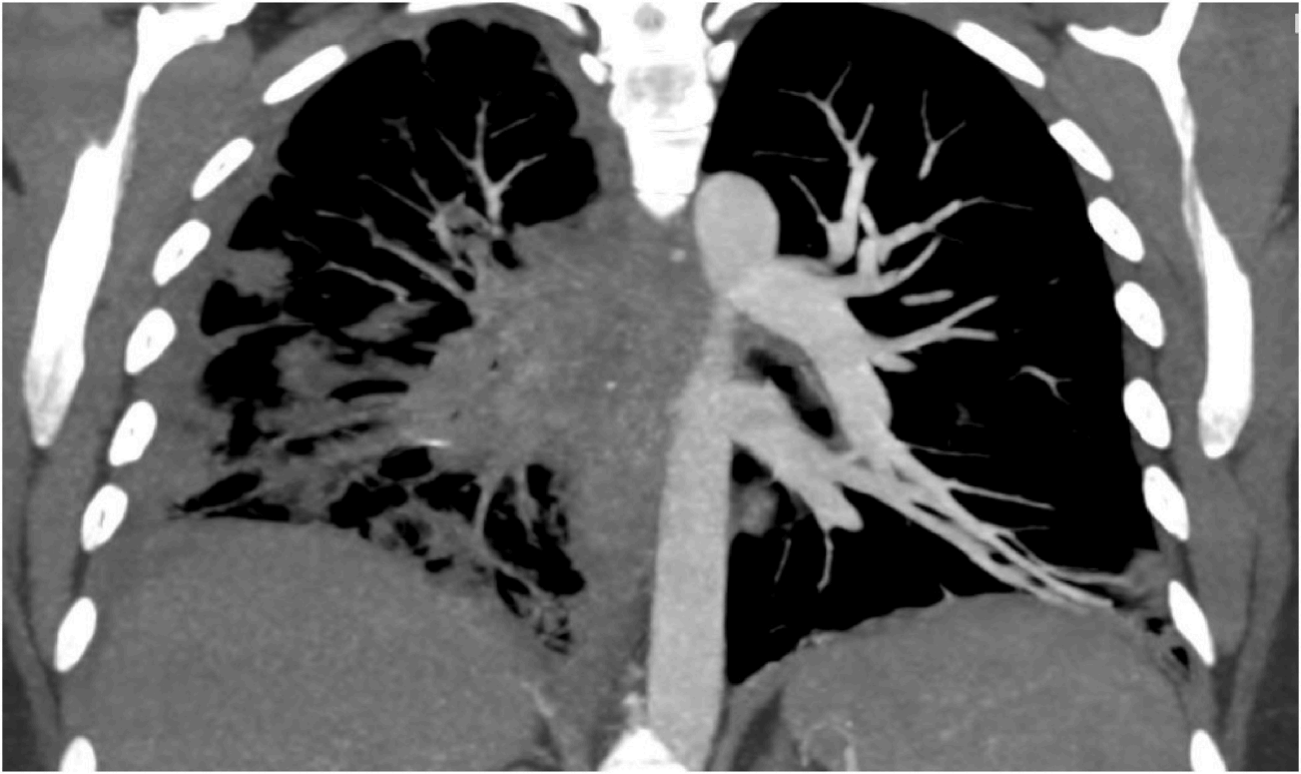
Coronal slice of computed tomography pulmonary angiography showing severe stenosis of the right pulmonary artery and mediastinal, hilar, and parenchymal fibrosis.
